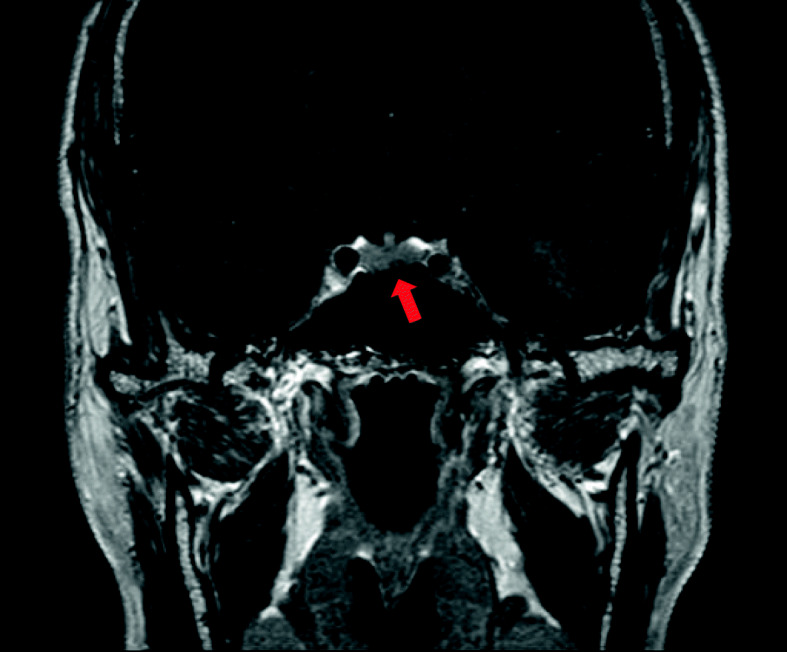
Sella MRI (coronal T1-weighted postcontrast) showing a 0.3-cm-sized, delayed enhancing lesion of the pituitary gland, suggestive of a pituitary microadenoma (red arrow)
